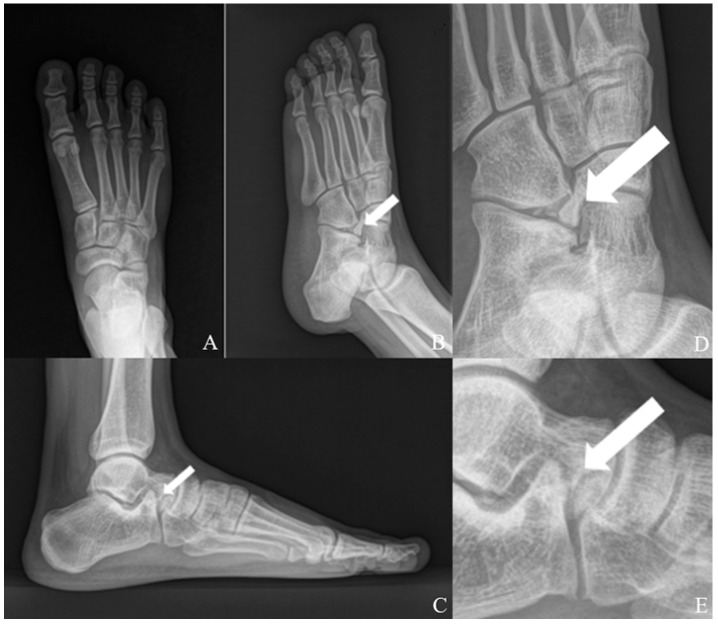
Preoperative plain radiographs of the left foot. (A) Anteroposterior, (B) oblique, and (C) lateral views demonstrate an accessory ossicle adjacent to the anterior process of the calcaneus (arrow). (D,E) Magnified views show a well-defined, smoothly marginated ossicle without features suggestive of an acute fracture fragment.
